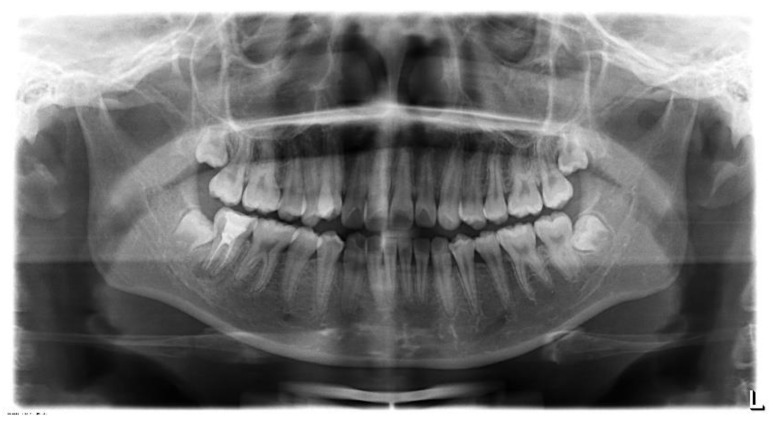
X-ray orthopantomography of the patient.
